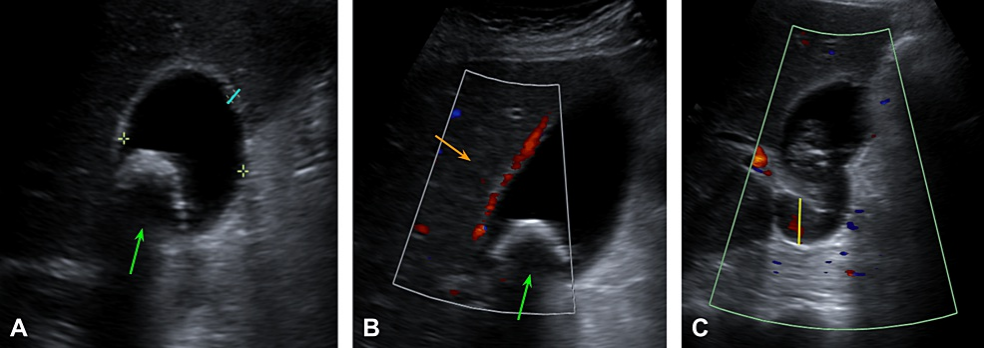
Liver and bile duct ultrasound Image A: Gallbladder lithiasis with a 2.8 cm stone (green arrow) and a 10 x 4 cm gallbladder with thickening of the walls up to 2.6 mm (blue line). Image B: Gallbladder lithiasis (green arrow) and gallbladder edema (orange arrow). Image C: Coledochus diameter of 6.6 mm (yellow line).
